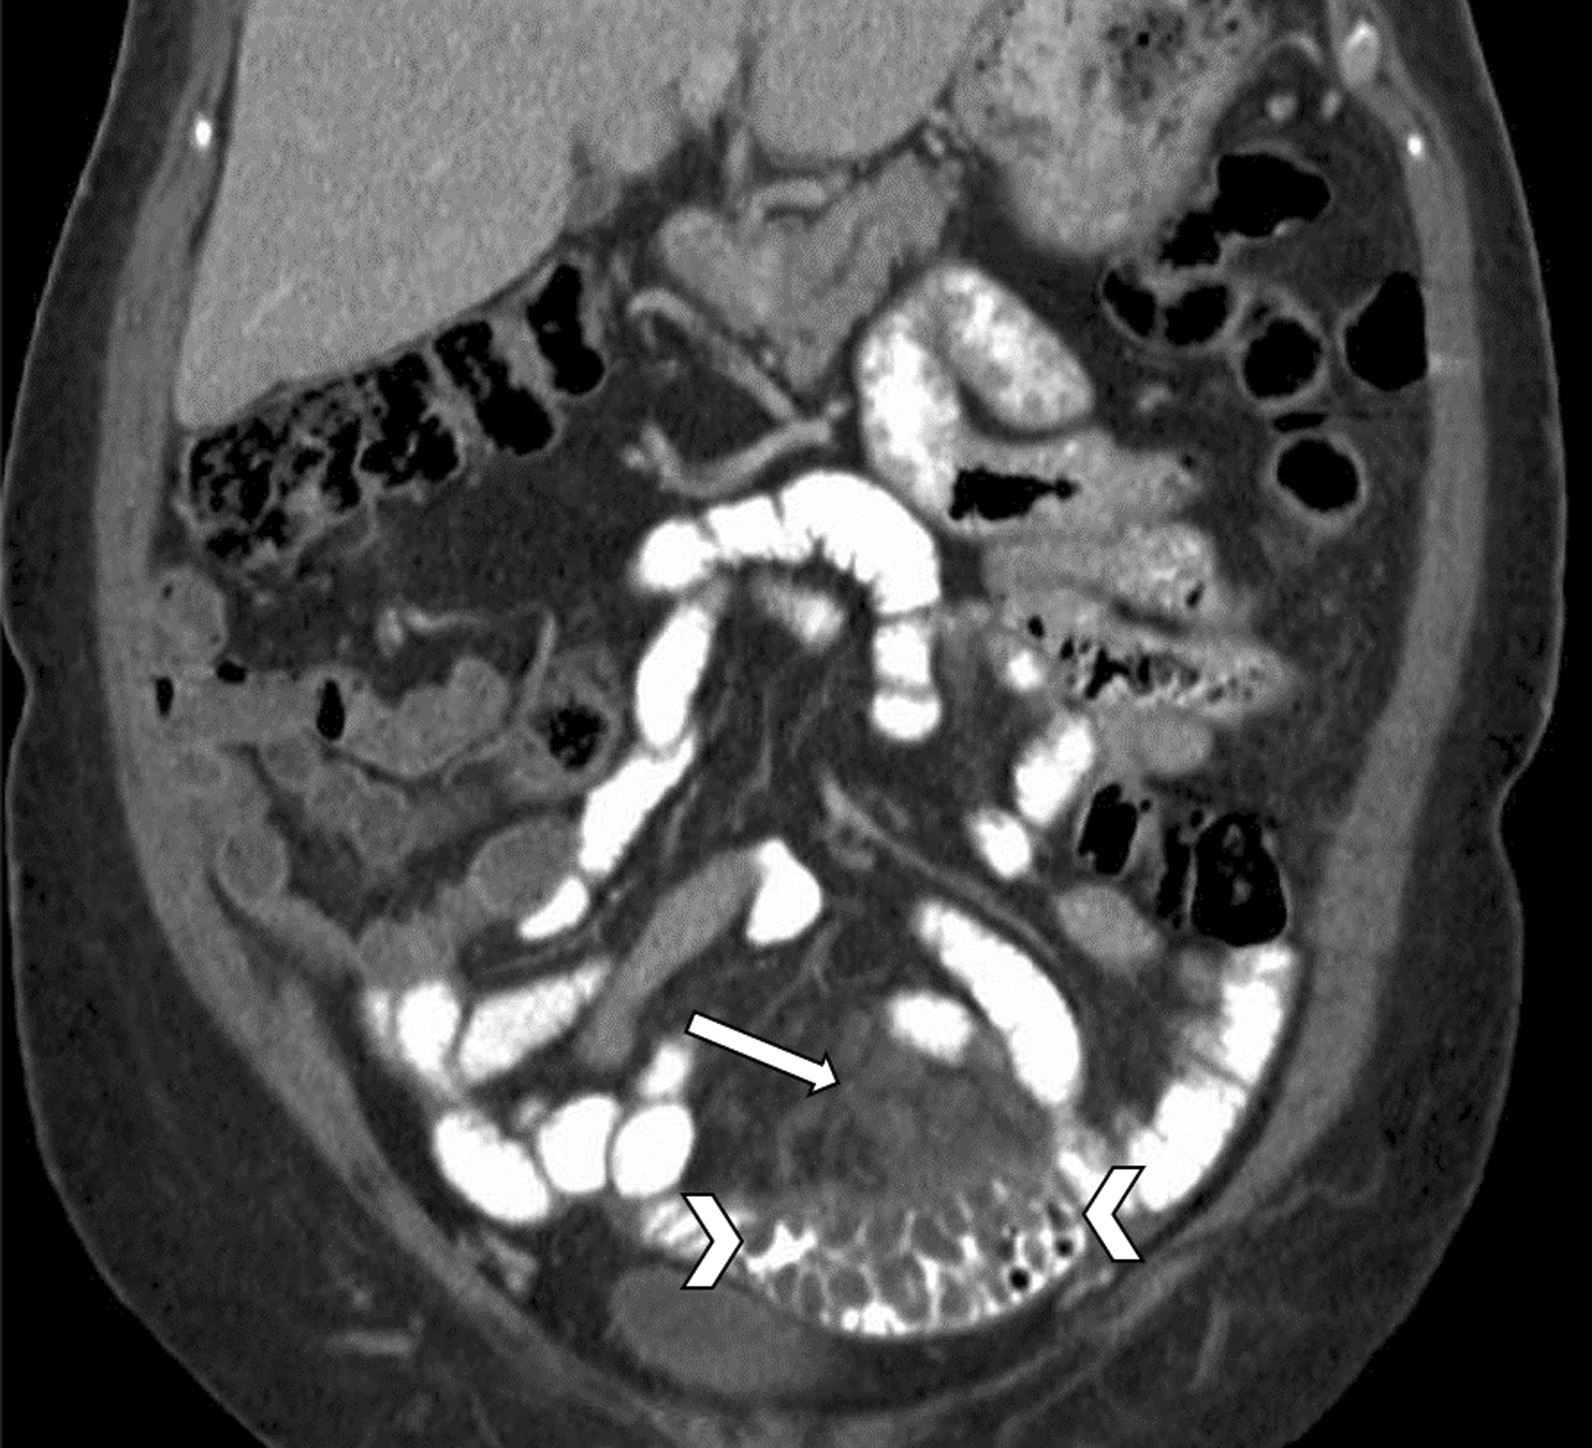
Abdominopelvic CT demonstrating an 8-cm segment of small bowel with low-density mural wall thickening (arrowheads) and multiple grape-like ovoid hypodense mesenteric fluid-containing structures (arrow)
